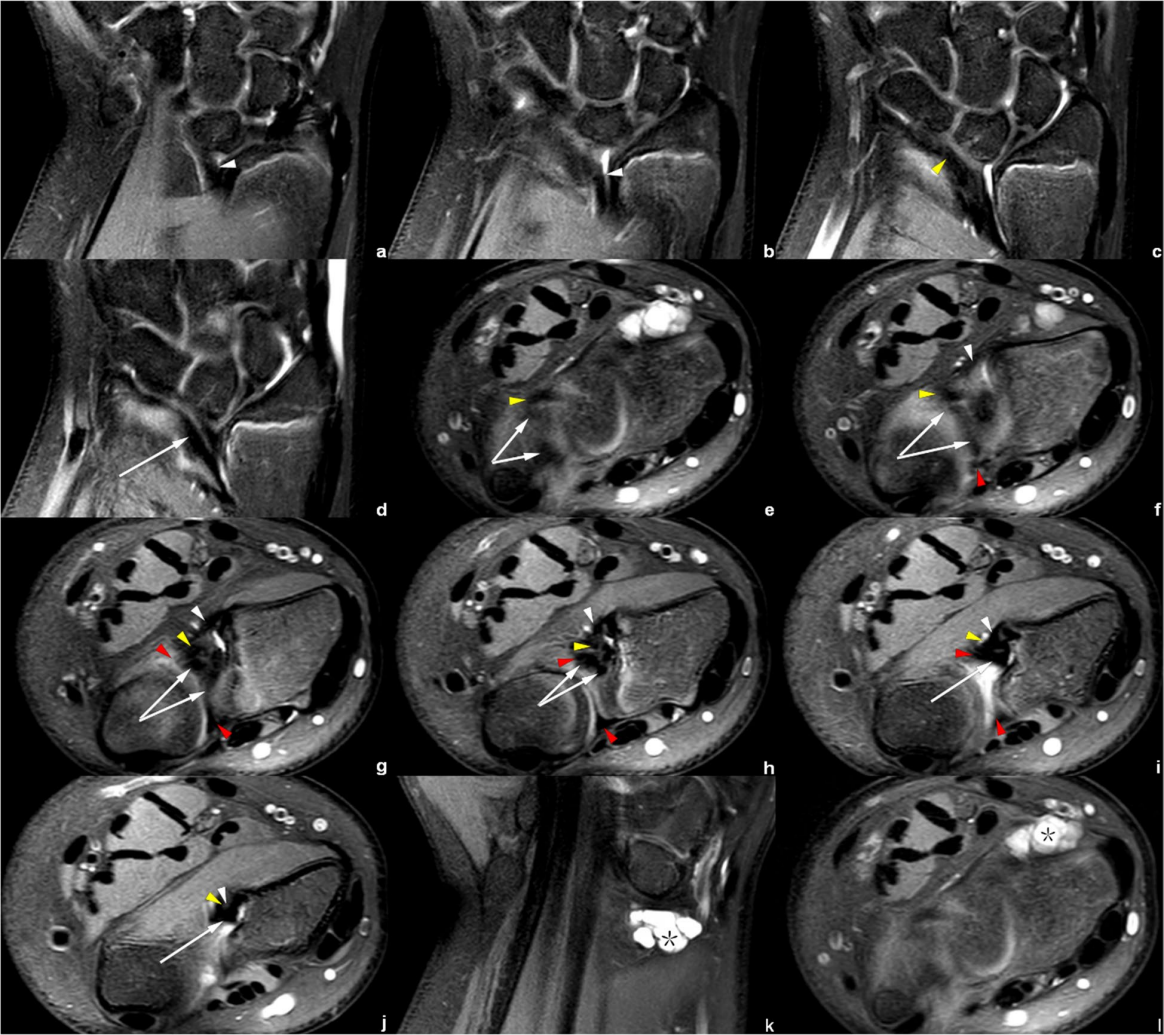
Right wrist MRI of the patient No. 0769. a–d Coronal PDWI-FS images show the VL (white arrowhead), RTL (yellow arrowhead), and CD (white arrow). e–j Axial PDWI-FS images show the CD (white arrow), volar and distal radioulnar ligament (red arrowhead), VL (white arrowhead), and RTL (yellow arrowhead). k–l Coronal and axial PDWI-FS images show a ganglion cyst. The lesion was detected by accident
